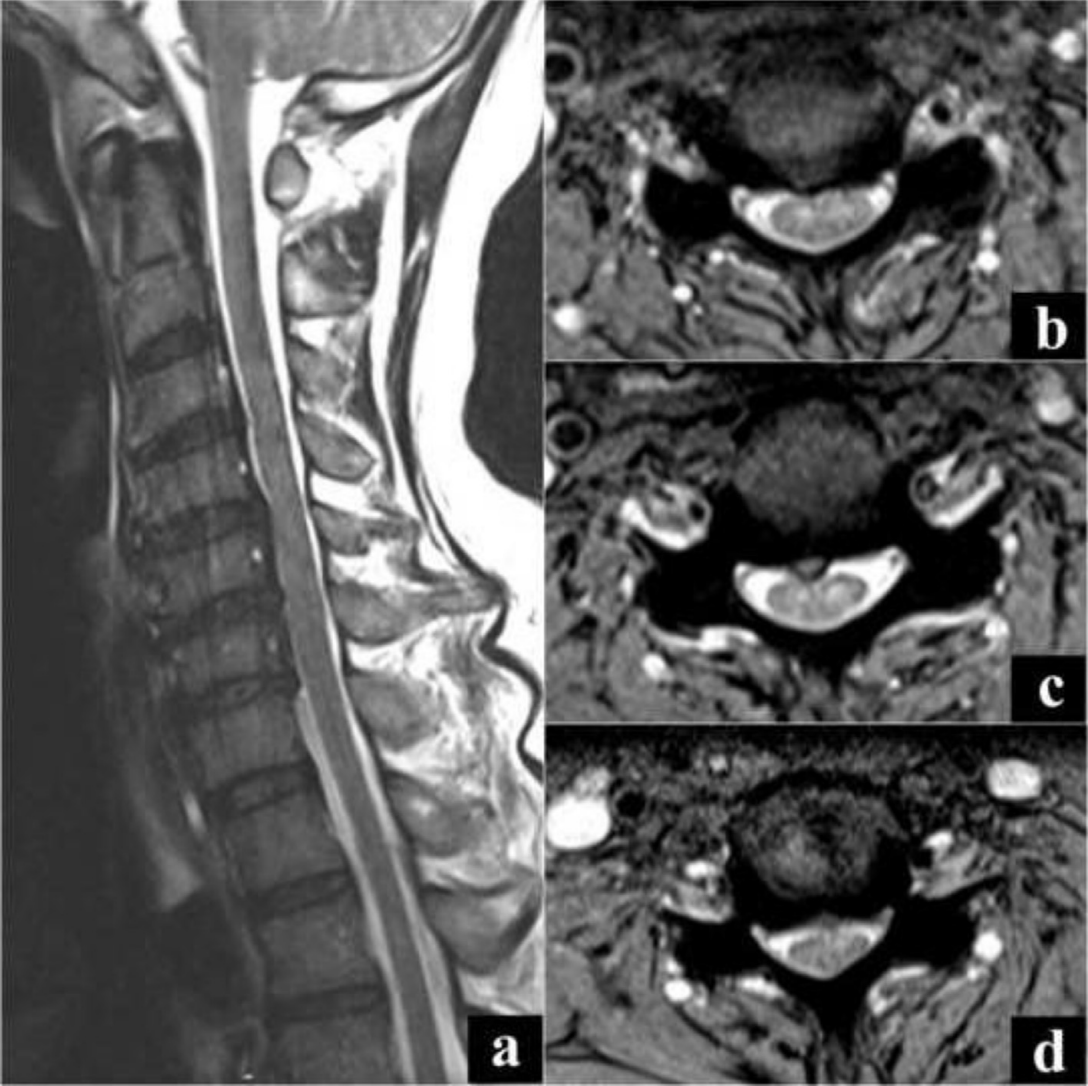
Second MR image showing mild cord signal changes on top of the C3–C6 canal stenosis seen earlier, suggestive of early myelomalacia. a) Sagittal cut image, b) Axial cut at C3–C4 level, c) Axial cut at C4–C5 level, d) Axial cut at C5–C6 level.
